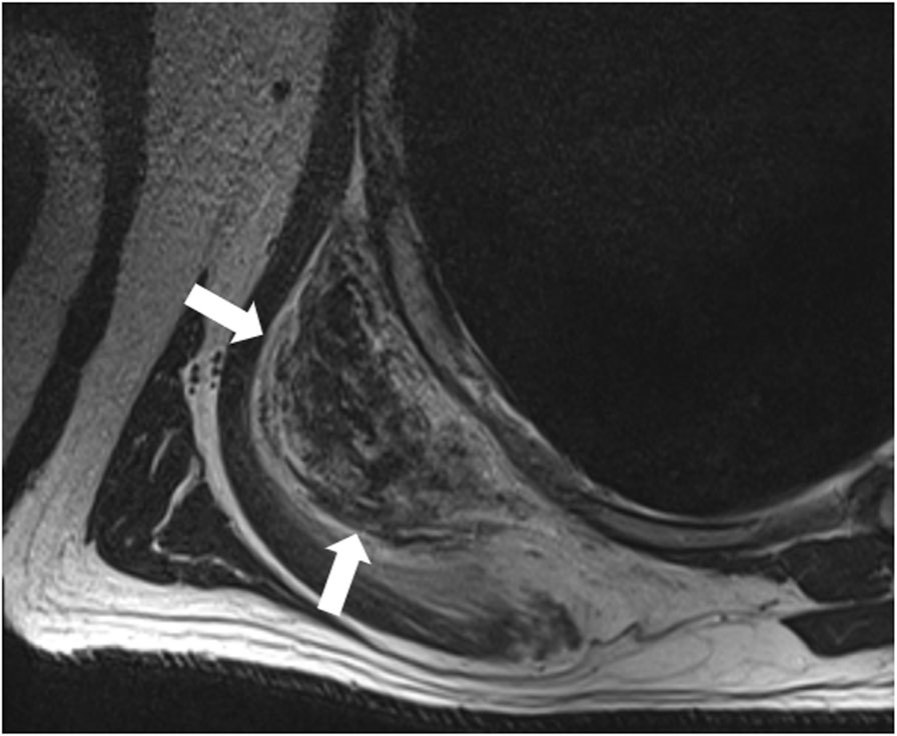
Axial T2-weighted turbo spin-echo (TSE) MR image showing a well-defined mass within the right infrascapular region (arrows) exhibiting multilayered appearance with internal fatty streaks consistent with elastofibroma
